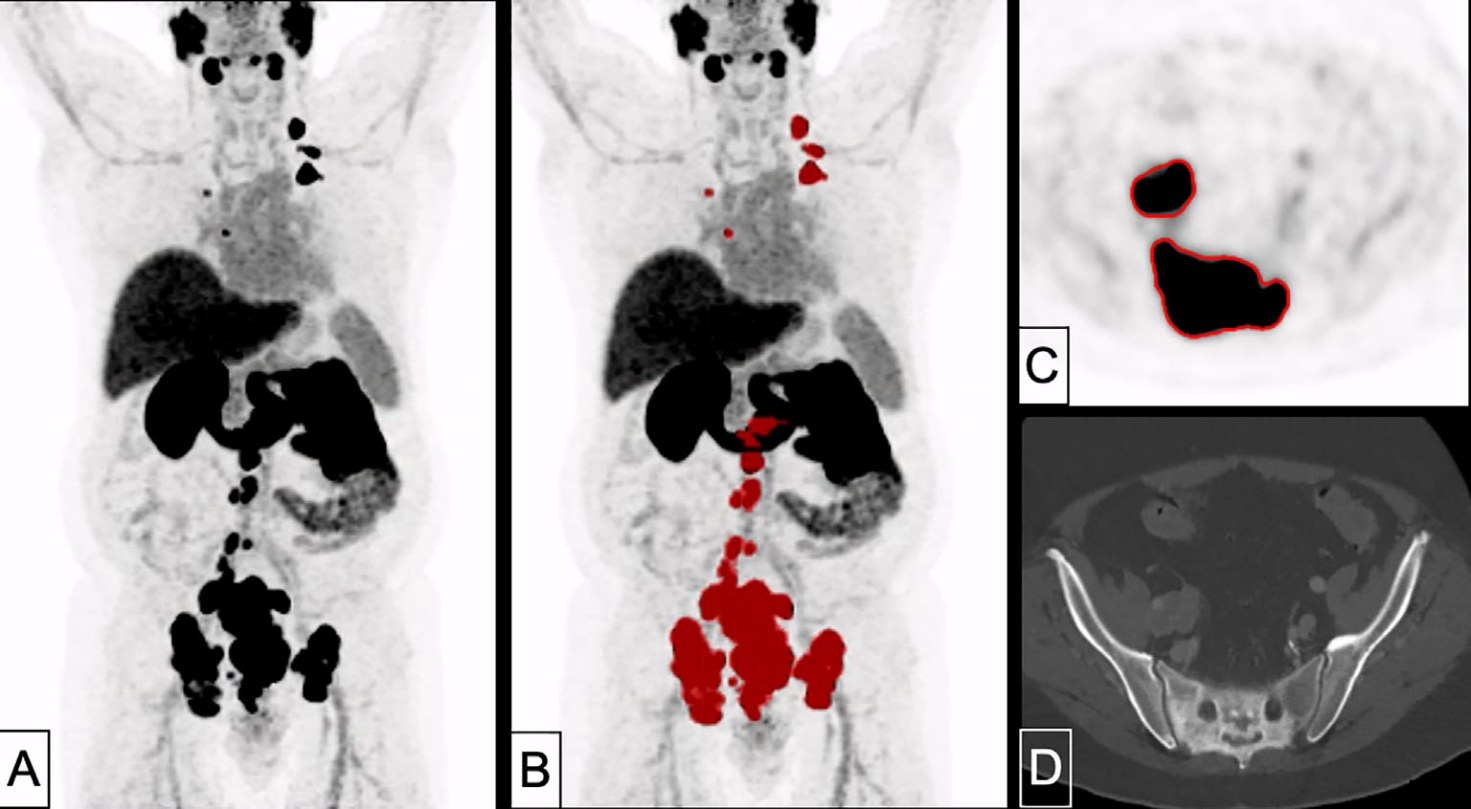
A 70 years-old patient with primary prostate cancer remnant with bone, pleura and lymph node metastases (PSA 78.0 ng/ml, Gleason 10). Tumor delineation using a cut-off of SUV 4.0 revealed a WTV of 586 ml. (A): MIP; (B) MIP + WTV (red color); (C) delineation of bone and lymph node metastasis on PET; (D) CT correlate (bone window).
